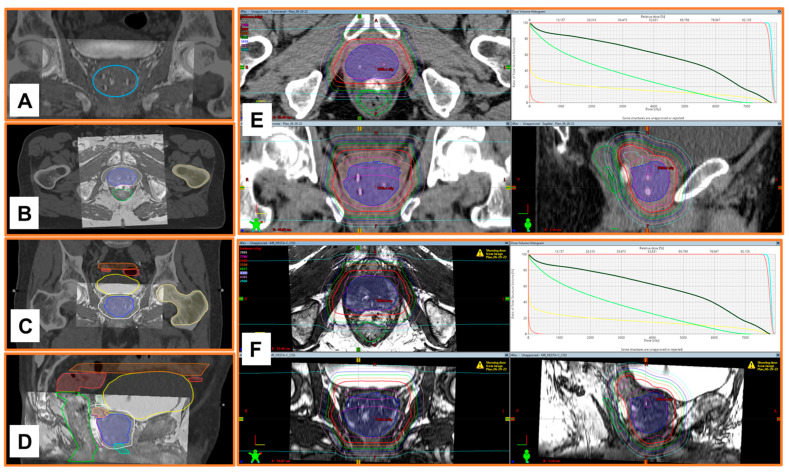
Use of NOVA markers for MRI/CT image fusion, contouring organs of interest, and generating radiation treatment plans. Pelvic MRI and CT images of a patient with prostate cancer were imported to the RayStation treatment planning system. Bony and soft tissue landmarks (specifically the prostate) were used to manually fuse axial CT and axial fully balanced steady state free precession (bSSFP) pulse sequence MR images. The positive NOVA fiducial marker signals from the CT and MR images were used to improve the fusion (panel ((A), inside the blue circle). The prostate, seminal vesicles, bladder, urethra, and rectum were contoured on the CT-MRI fused images (axial, panel (B); coronal, panel (C); sagittal, panel (D)). Panels (E,F) show proton radiation treatment plans generated based on CT only (E) and on MRI/CT image fusion (F).
